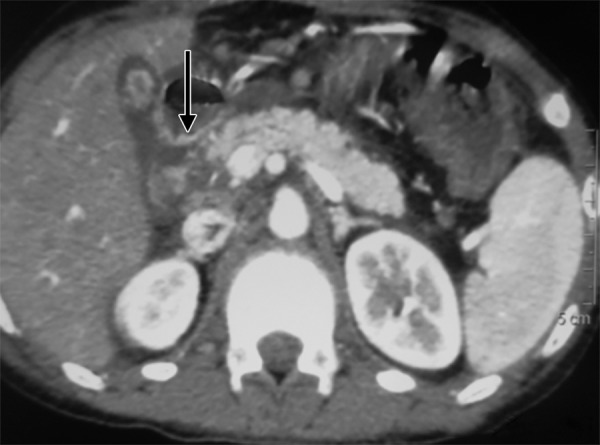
Contrast enhanced computed tomography scan showing an ill-defined collection in the Morrison’s pouch and mild free fluid in the peritoneal cavity
